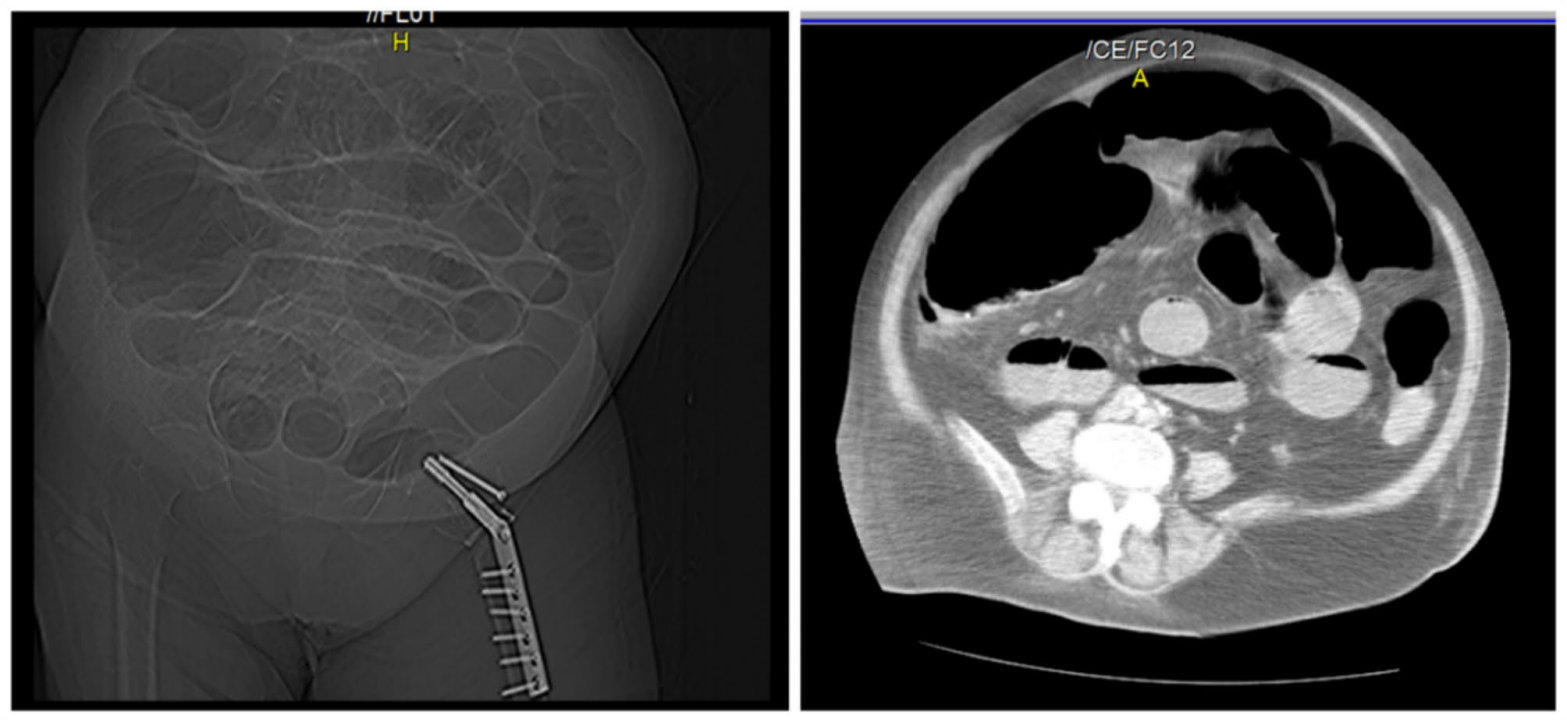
Abdominal CT that showed a gross dilated in colons, up to 92.4 mm at the cecum level
